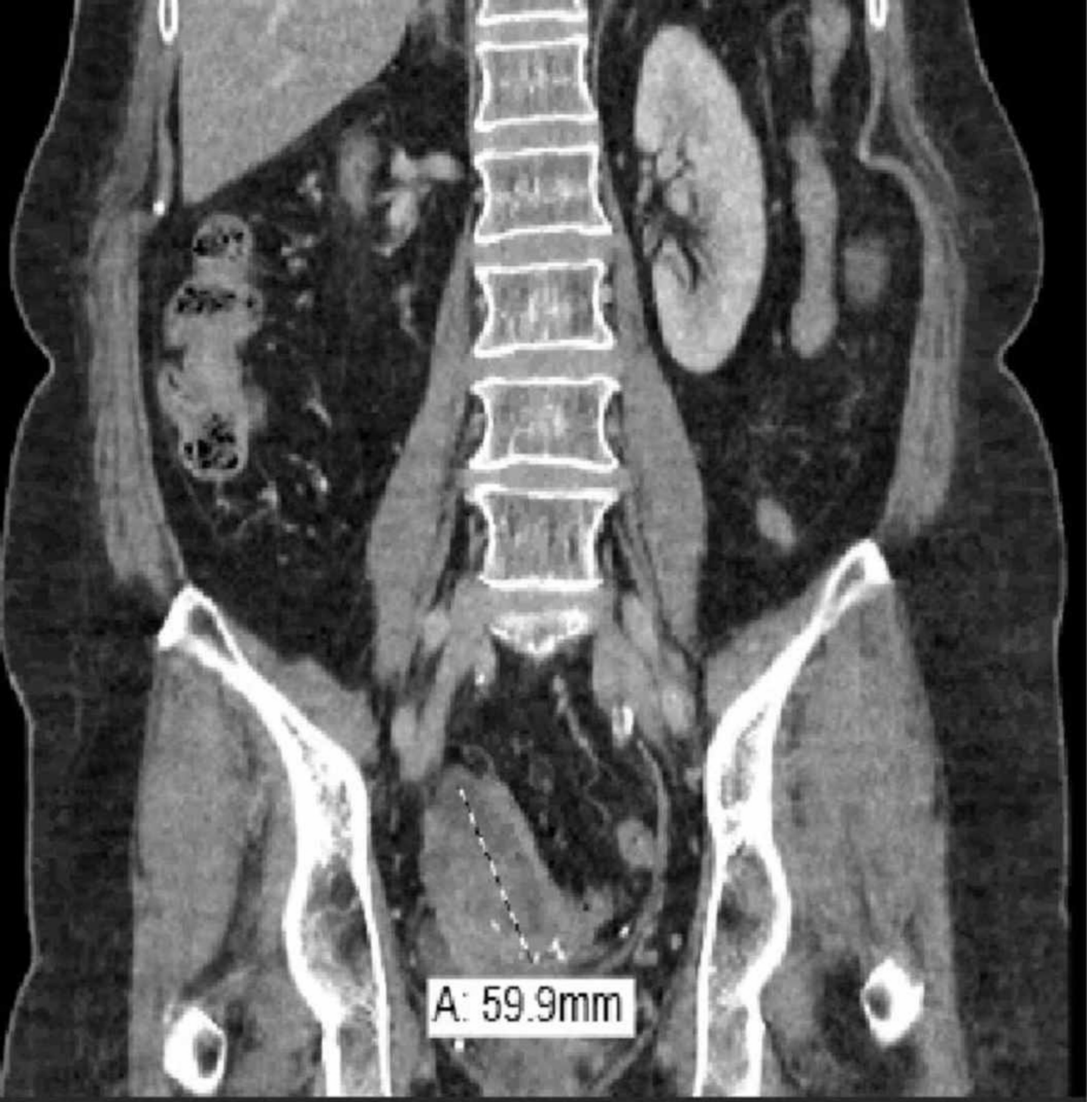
Coronal view of CT abdomen and pelvis reveals a large solid/cystic pelvic mass surrounding the right ureter
